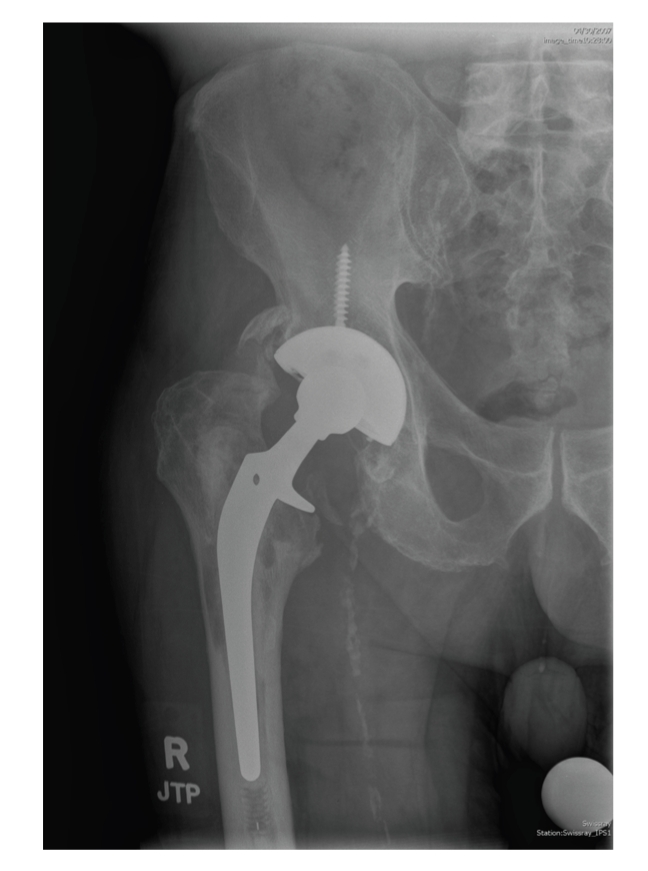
Anteroposterior X-rays of right hip showing lucencies around femoral and acetabular prosthesis.
